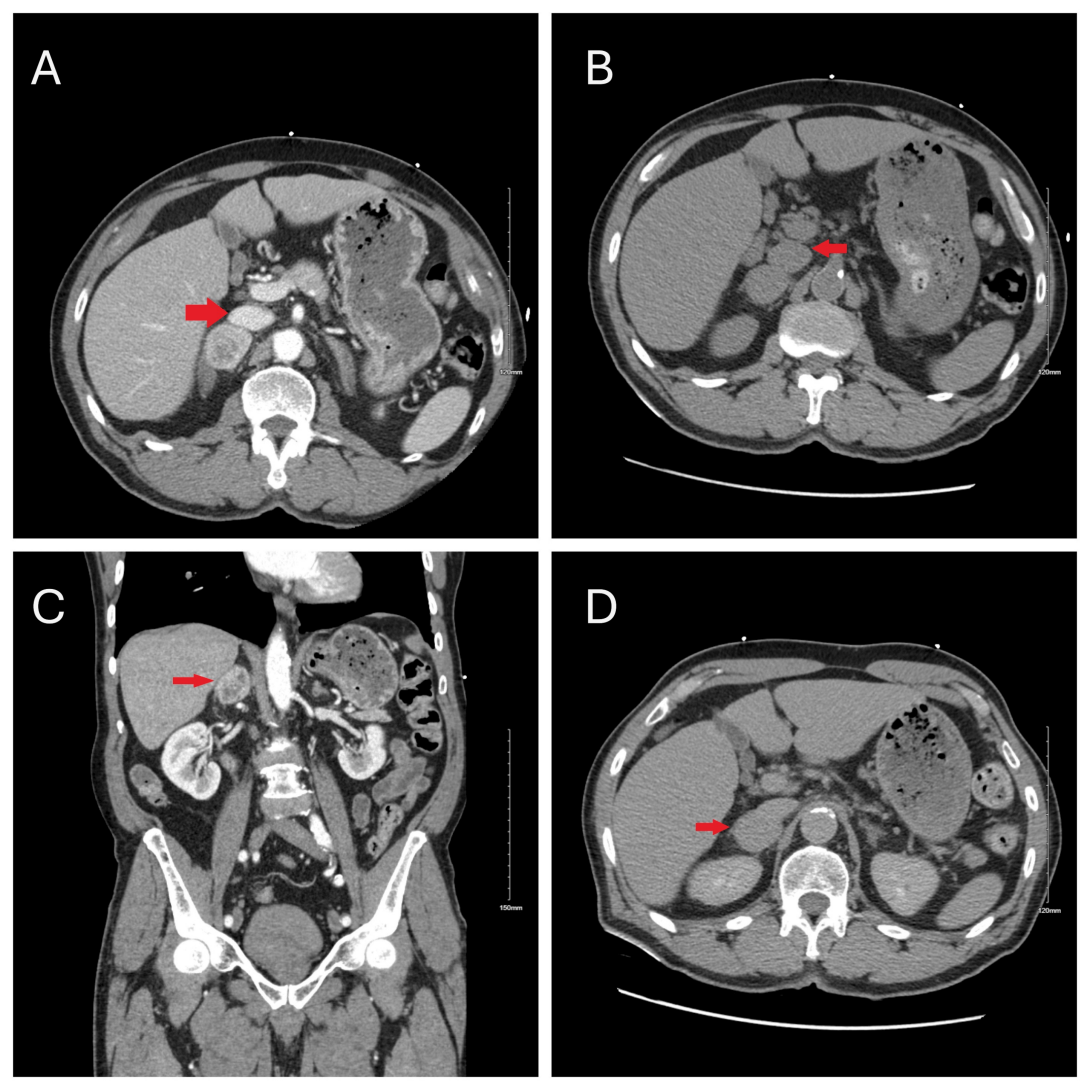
Computed tomography (CT) scan of the abdomen demonstrating a right adrenal mass (red arrow).
